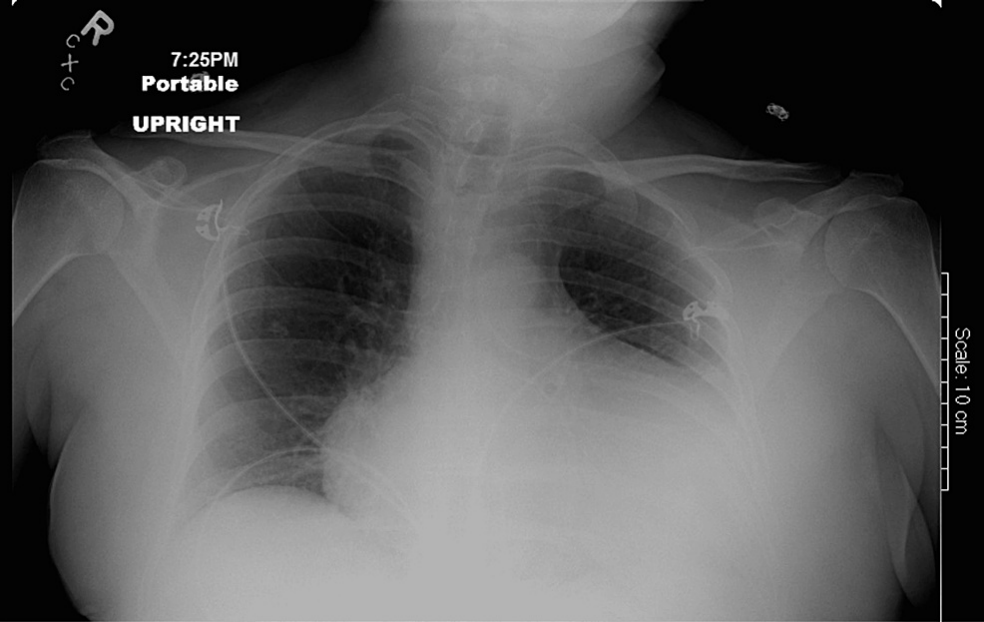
Chest X-ray revealed a classic “water bottle” appearance of the cardiac silhouette suggestive of pericardial effusion.
